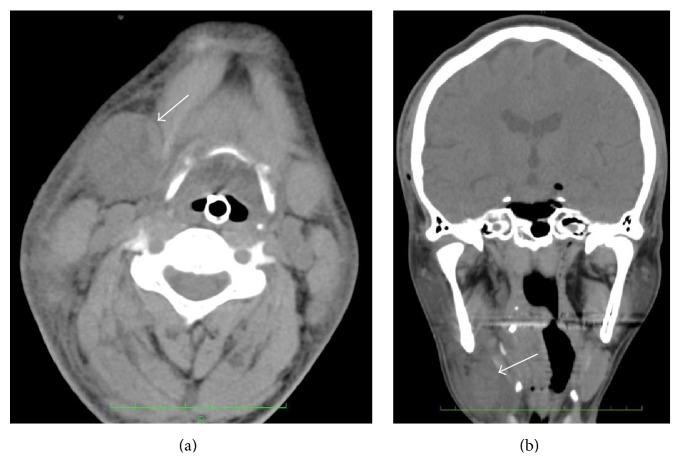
Axial (a) and coronal (b) images of computed tomography 1 hour after operation showing swelling of the right submandibular gland and the associated edematous tissue (arrow).
